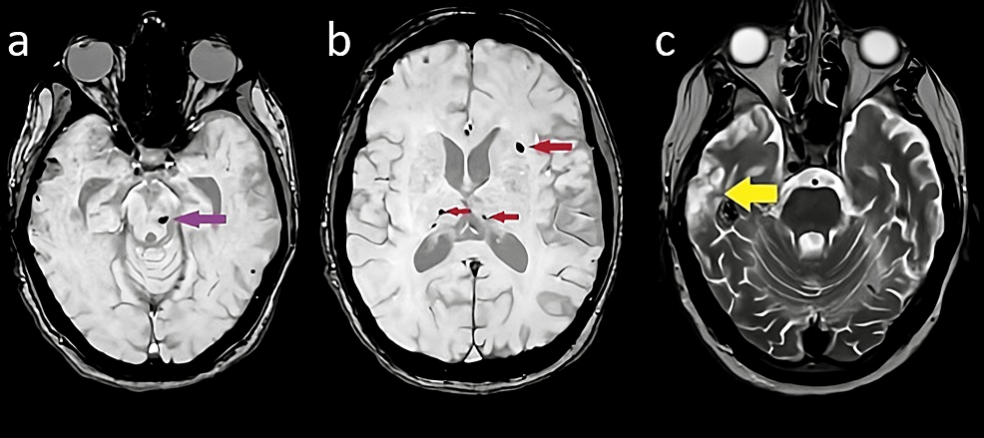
Brain magnetic resonance imaging a, b: Susceptibility weighted imaging (SWI) sequences - showing the presence of multiple hemosiderin deposits (purple and red arrows) - are compatible with a diffuse axonal damage. c: T2-weighted sequence, showing the result of a temporal contusion (yellow arrow) due to the road accident.
